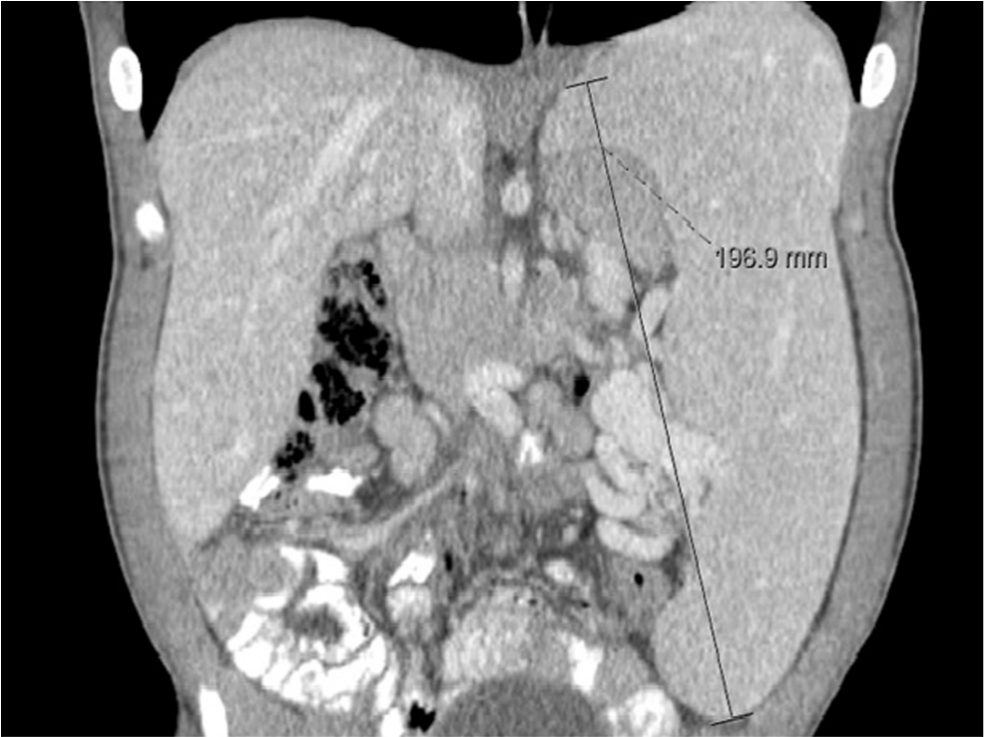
Abdominal CT-scan showing massive hepatosplenomegaly seen in our patient before treatment.
